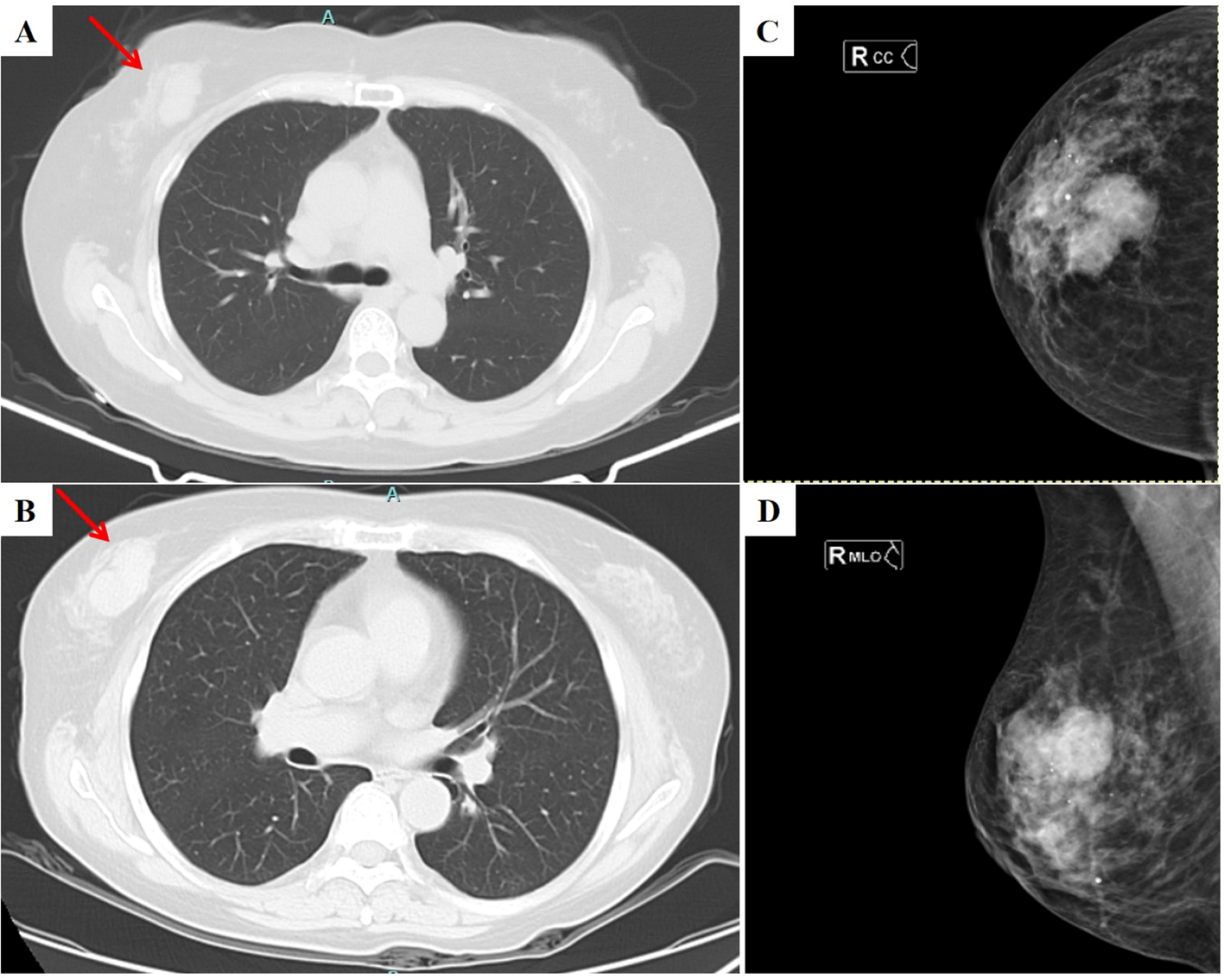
CT and MMG images of the tumor. (A) Chest CT showed an irregular solid mass in the upper quadrant of the right breast (this image was taken on October 26, 2023. The location of the lesion is indicated by the red arrow); (B) Chest CT showed an irregular solid mass in the upper quadrant of the right breast that was larger than before (this image was taken on May 29, 2024. The location of the lesion is indicated by the red arrow); (C) The head and tail positions of the MMG show an irregular mass shadow in the middle and upper part of the right breast (this image was taken on June 11, 2024); (D) The internal and external oblique position of MMG shows an irregular mass shadow in the middle and upper part of the right breast (this image was taken on June 11, 2024); CT, computed tomography; MMG, mammography.
